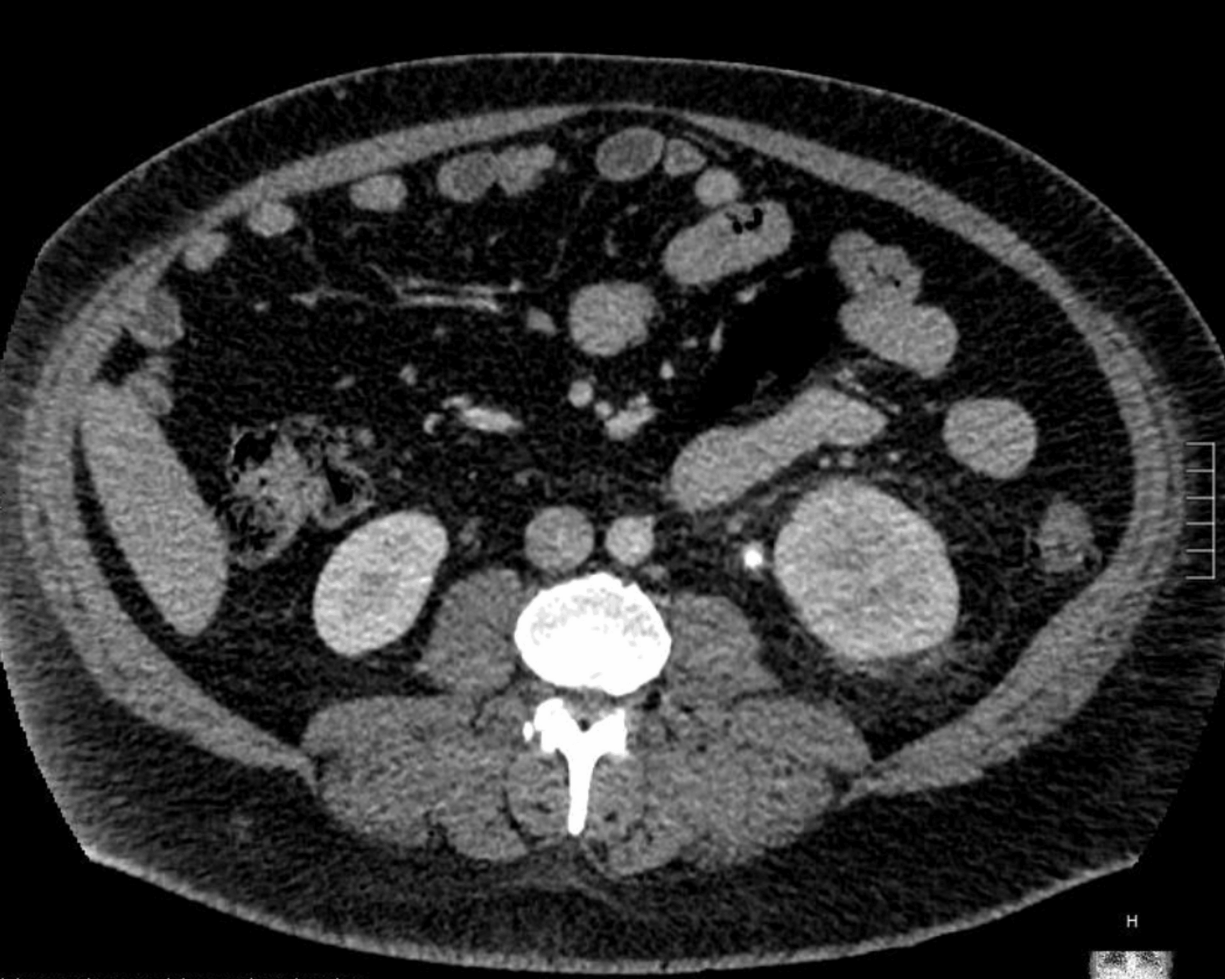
CT abdomen/pelvis showing proximal left ureteral stone, moderate left-sided hydronephrosis, and left perirenal stranding.
